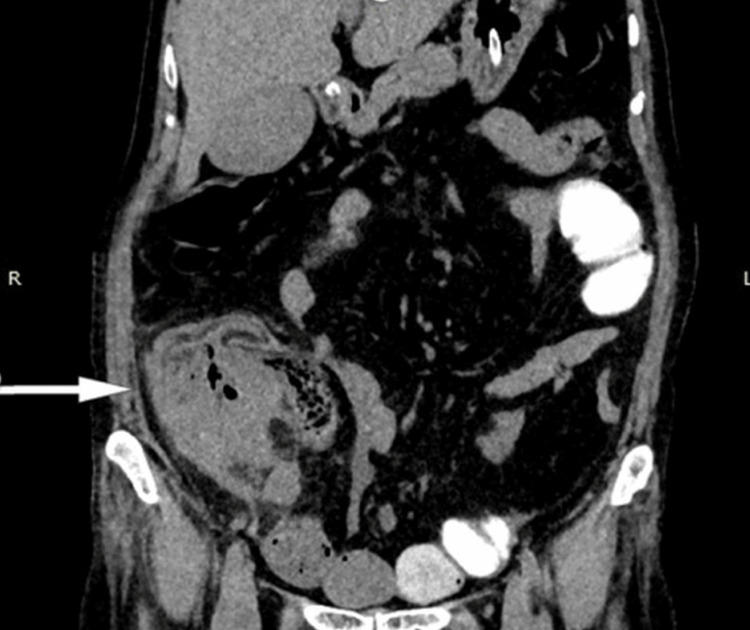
Contrast-enhanced CT showing appendicular lump formation following base of appendix perforation.
